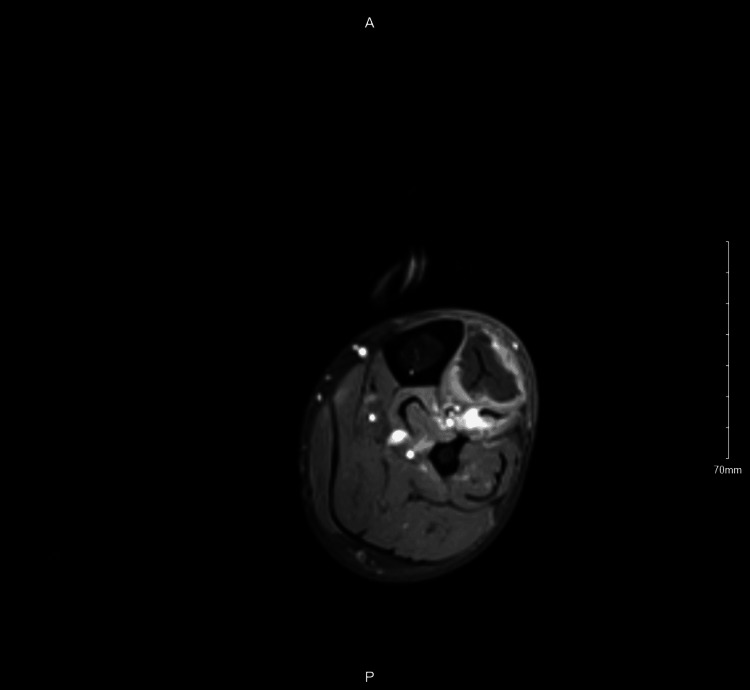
MRI of the anterior compartment of the left lower limb showing tibialis anterior mildly enlarged, and it appears hypointense.
